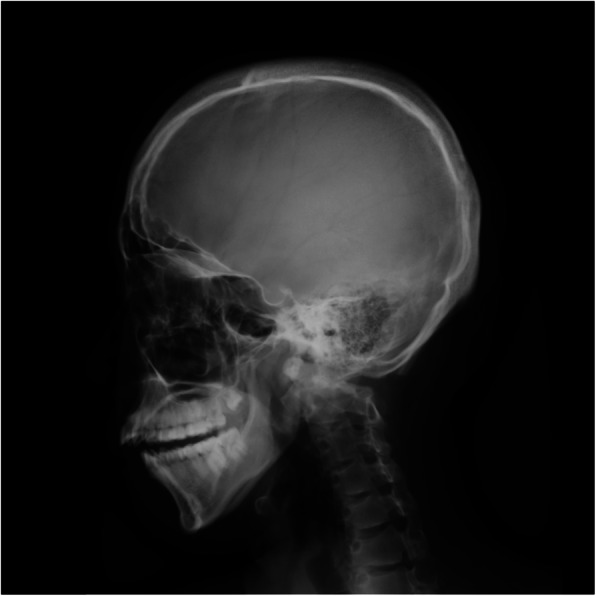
A lateral X-ray image of the head showing micrognathia
